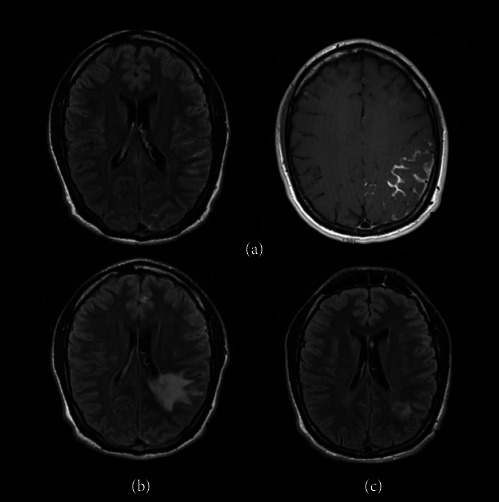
Evolution of CNS lesions in MRI. (a) Pretreatment: thickened left frontal, parietal, occipital cortex and leptomeningeal enhancement. (b) Post 3 month treatment: increased thickness and density in left parietal white matter, indicating cerebritis and edema. (c) Post 1 month corticosteroid reintroduction: overall improvement, residual left parietal white matter edema.
